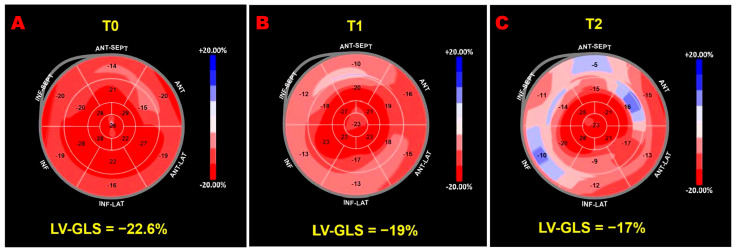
Speckle-tracking echocardiography–derived LV-GLS bull’s-eye plots obtained in a patient enrolled in the present study who developed ICI-related cardiotoxicity. (A) Normal LV-GLS bull’s-eye plot at baseline (T0). (B) Mild impairment of the LV-GLS bull’s-eye plot at the 1-month follow-up (T1). (C) Moderate impairment of the LV-GLS bull’s-eye plot at the 3-month follow-up (T2). ICI, immune checkpoint inhibitors; LV-GLS, left ventricular global longitudinal strain.
